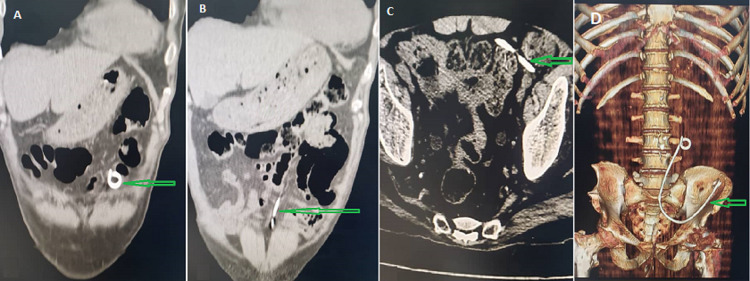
abdominal CT scan showing: A) distal coil of double J stent in the peritoneal cavity (arrow); B) double J stent in the peritoneal cavity (arrow); C) proximal coil of double J stent in the peritoneal cavity (arrow); D) helical computed tomography shows the DJ stent's total displacement in the peritoneal cavity (arrow)
